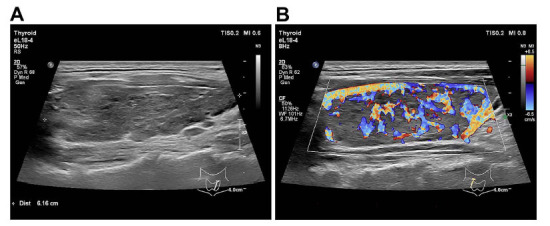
Thyroid ultrasonography. (A). Slight swelling in lobe. (B). Rich blood flow in parenchyma.
